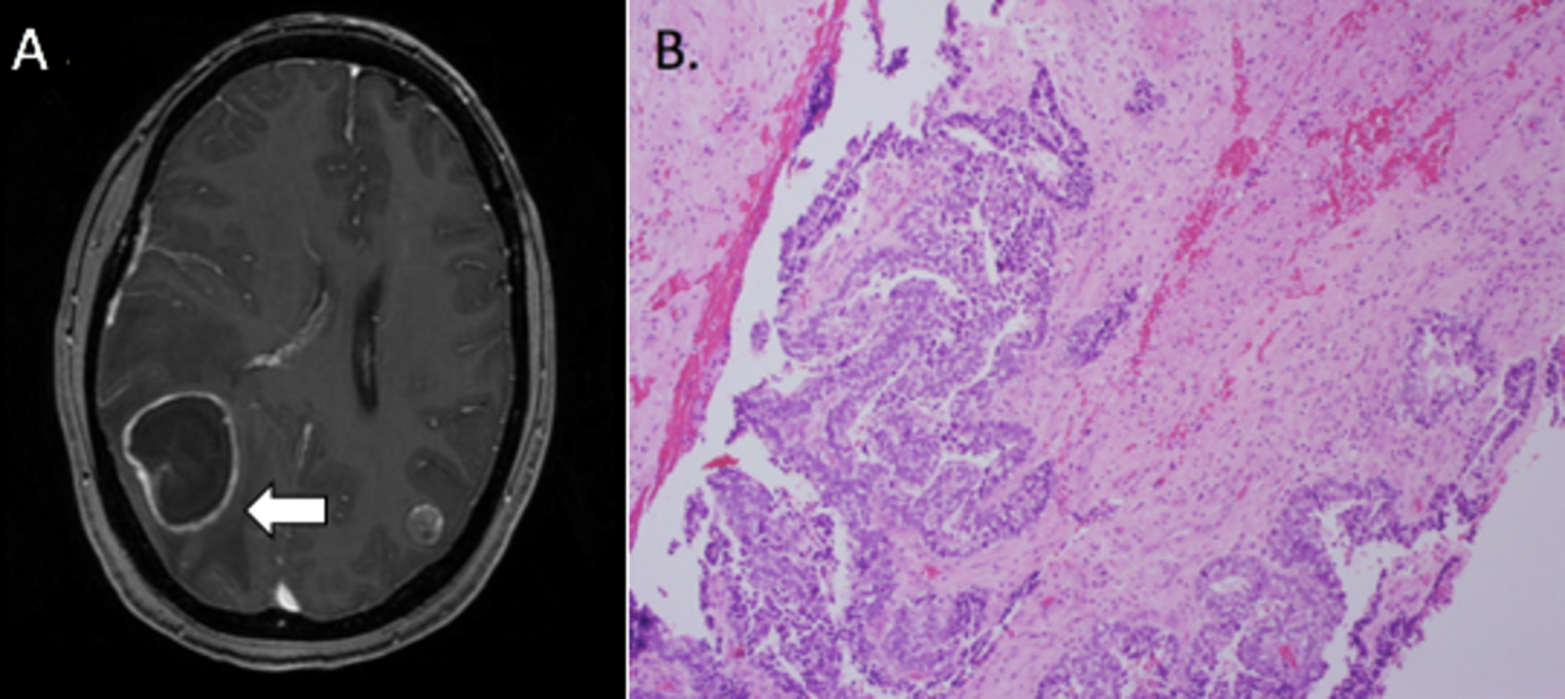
Case 1: brain metastasis (A) Brain magnetic resonance imaging at the time of brain metastasis with right 4 x 3.4 x 3.7-cm temporo-occipital cavitary lesion, left parieto-occipital 1.0 x 1.1 x 1.0-cm lesion, vasogenic edema, and right to left midline shift. (B) Biopsy from the brain metastasis consistent with classic biphasic pulmonary blastoma.
